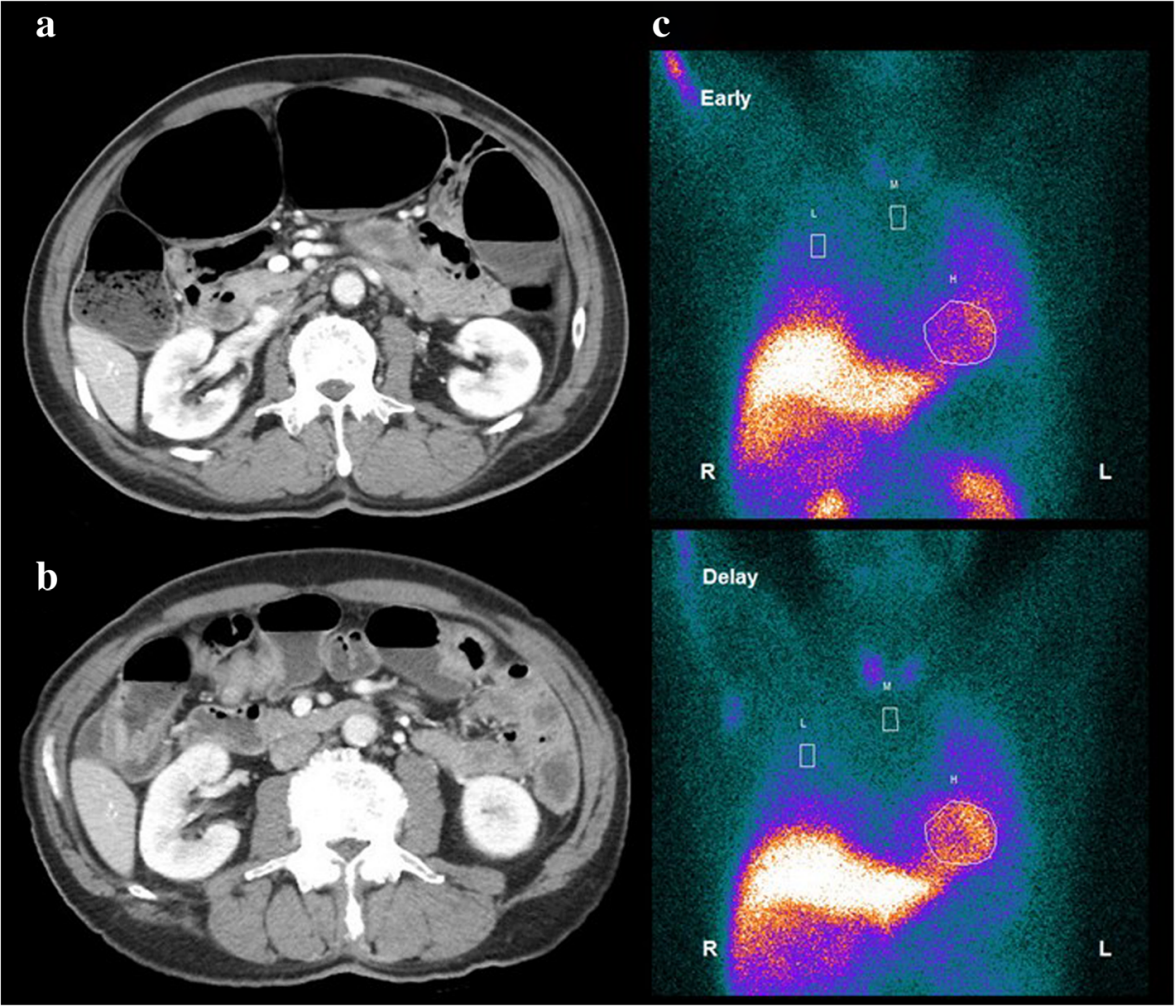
Abdominal contrast-enhanced computed tomography 13 days (a) and 83 days (b) after admission and ^123^I-metaiodobenzylguanidine (MIBG) myocardial scintigraphy 43 days after admission (c). Abdominal contrast-enhanced computed tomography showed marked colonic distention (a), and abdominal distention disappeared (b). The early and delayed heart/mediastinum (H/M) ratio of MIBG was 2.9 and 4.0, respectively (c). The washout ratio was 20.1%
